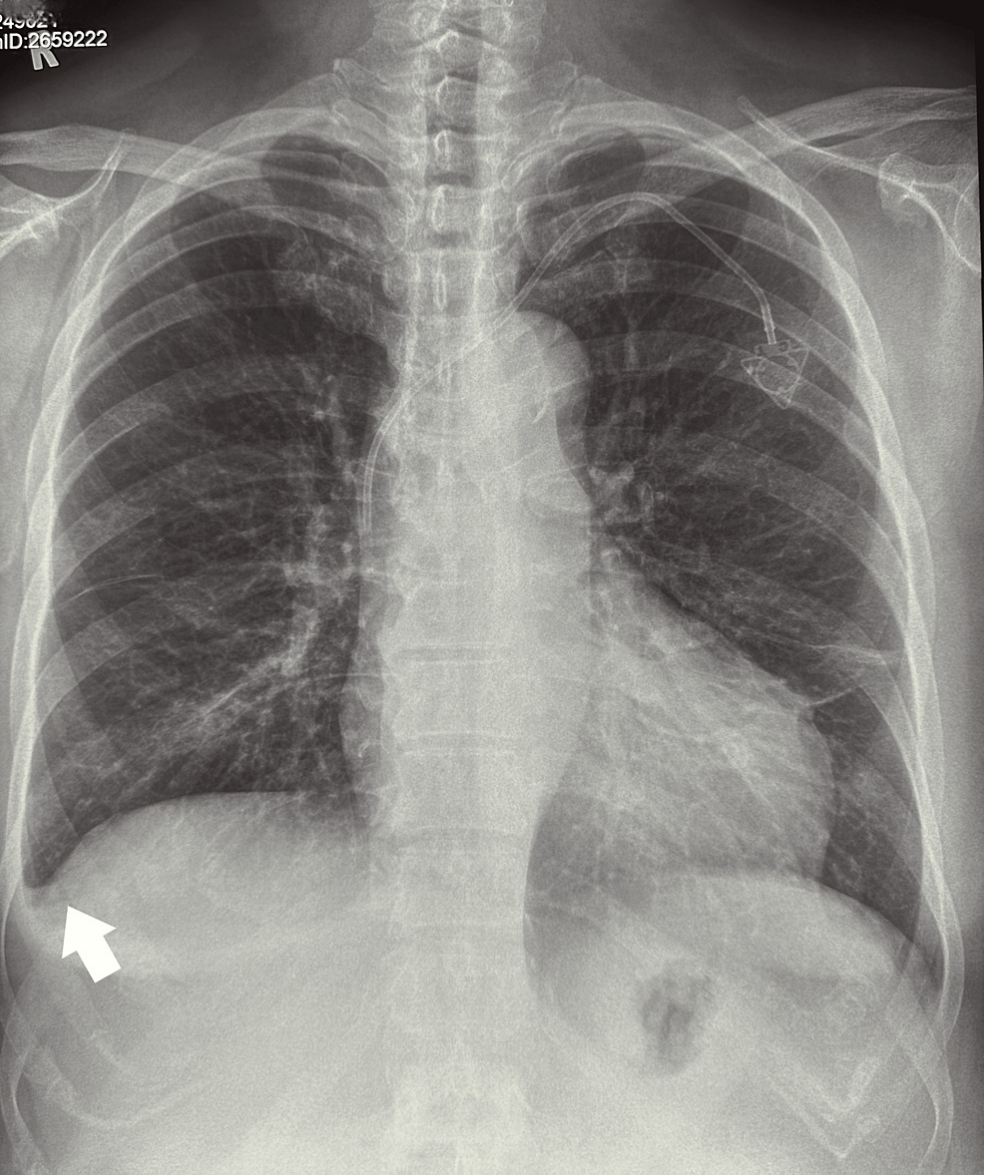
Chest radiograph Mild right lower zone infiltrates, linear density over the lateral left lower zone indicative of atelectasis, and blunting of the right costophrenic angle (white arrow) are indicative of pleural effusion. A chemo port is visible in the left side of the chest.
